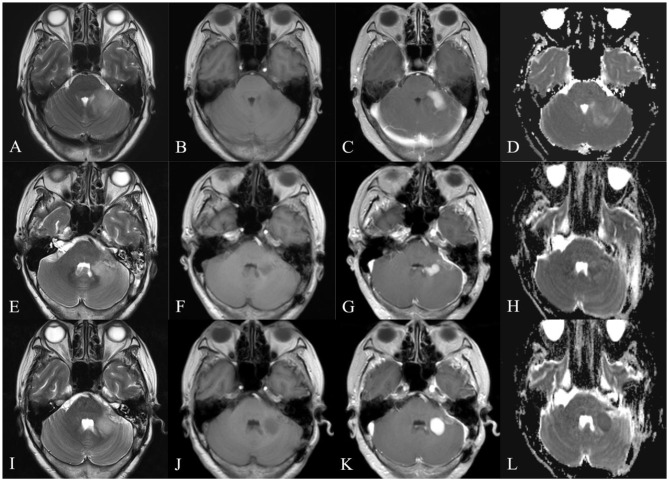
A 54-year-old female with relapsed/refractory PCNSL. Pretreatment MRI images (A-D). A solitary lesion was located in the left cerebellar peduncle, demonstrating mildly hyperintense signal on T2WI (A), hypointense signal on T1WI (B) and marked enhancement on CE-T1WI (C). The corresponding ADC map revealed rADC_min−pre_ of 0.885 and rADC_mean−pre_ of 1.159 (D). MRI images after a cycle of chemotherapy showed reduction in size compared to previous imaging (E-G). The corresponding ADC map demonstrated decreased signal intensity, with rADC_min−post_ of 0.747, rADC_mean−post_ of 1.012, rADC_min−change_ of -0.138, and rADC_mean−change_ of -0.147 (H). MRI images after the end of the whole chemotherapy exhibited interval enlargement in volume (I-L)
